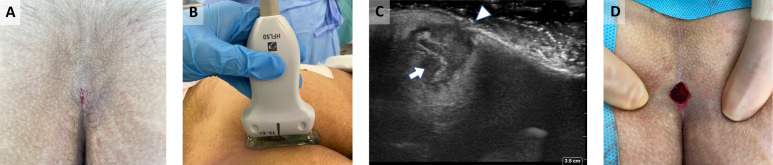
Infected pilonidal cyst. A)Natal cleft with small opening. B)Using high frequency linear probe with a layer of gel to scan the natal cleft area. C)Ultrasonographic sagittal view showing the pilonidal cyst and hair within (arrow) and the single pit (arrow head). No craniocaudal extension. D)Post excision.
